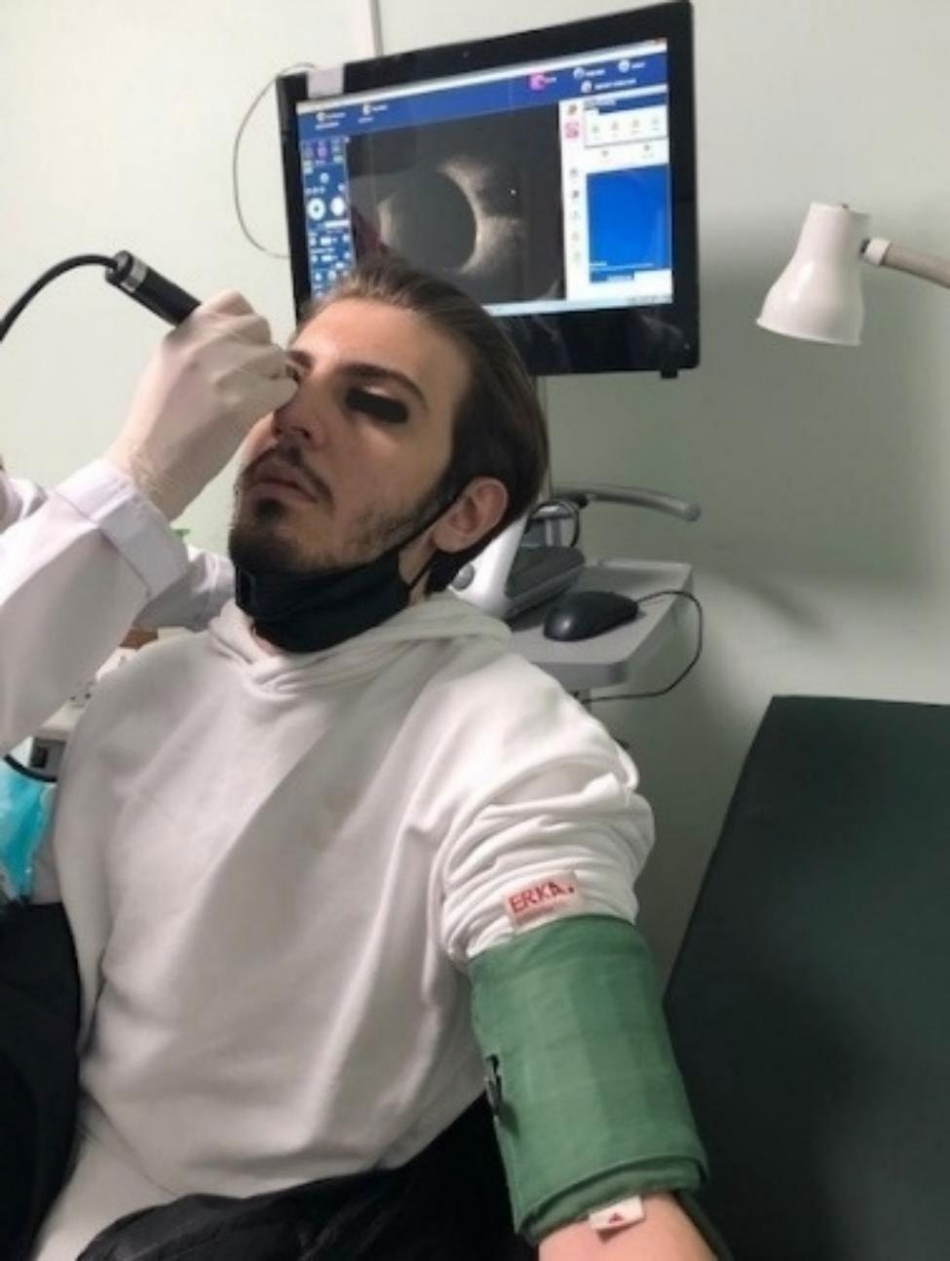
Measurement of optic nerve sheath diameter ultrasonographically
